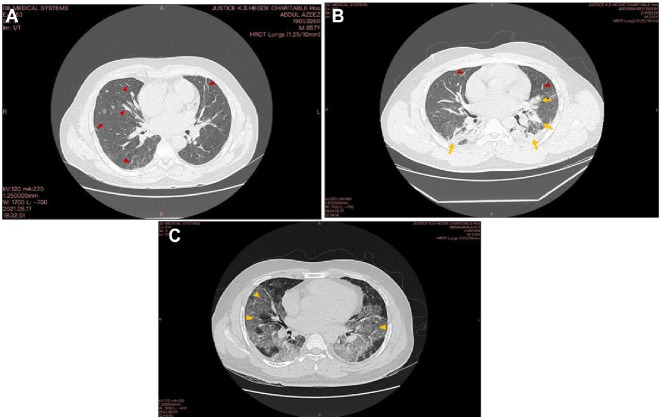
Representative images of axial high-resolution chest CT of the mild, moderate, and severe conditions of a diagnosed COVID-19 patient with CO-RAD 6 (A) indicates the axial HRCT showing bilateral fibrotic bands (red arrowheads) with predominantly peripheral distribution with a CT-SS of 6/25 (mild). (B) indicates the axial HRCT showing consolidations in both the lung fields (yellow arrows) and fibrotic bands (red arrowheads) with predominantly peripheral distribution with a CT-SS of 15/25 (moderate). (C) indicates axial HRCT showing bilateral patchy ground glass opacities (yellow arrowheads) with predominantly peripheral and peribronchovascular distribution with a CT-SS of 21/25 (severe).
